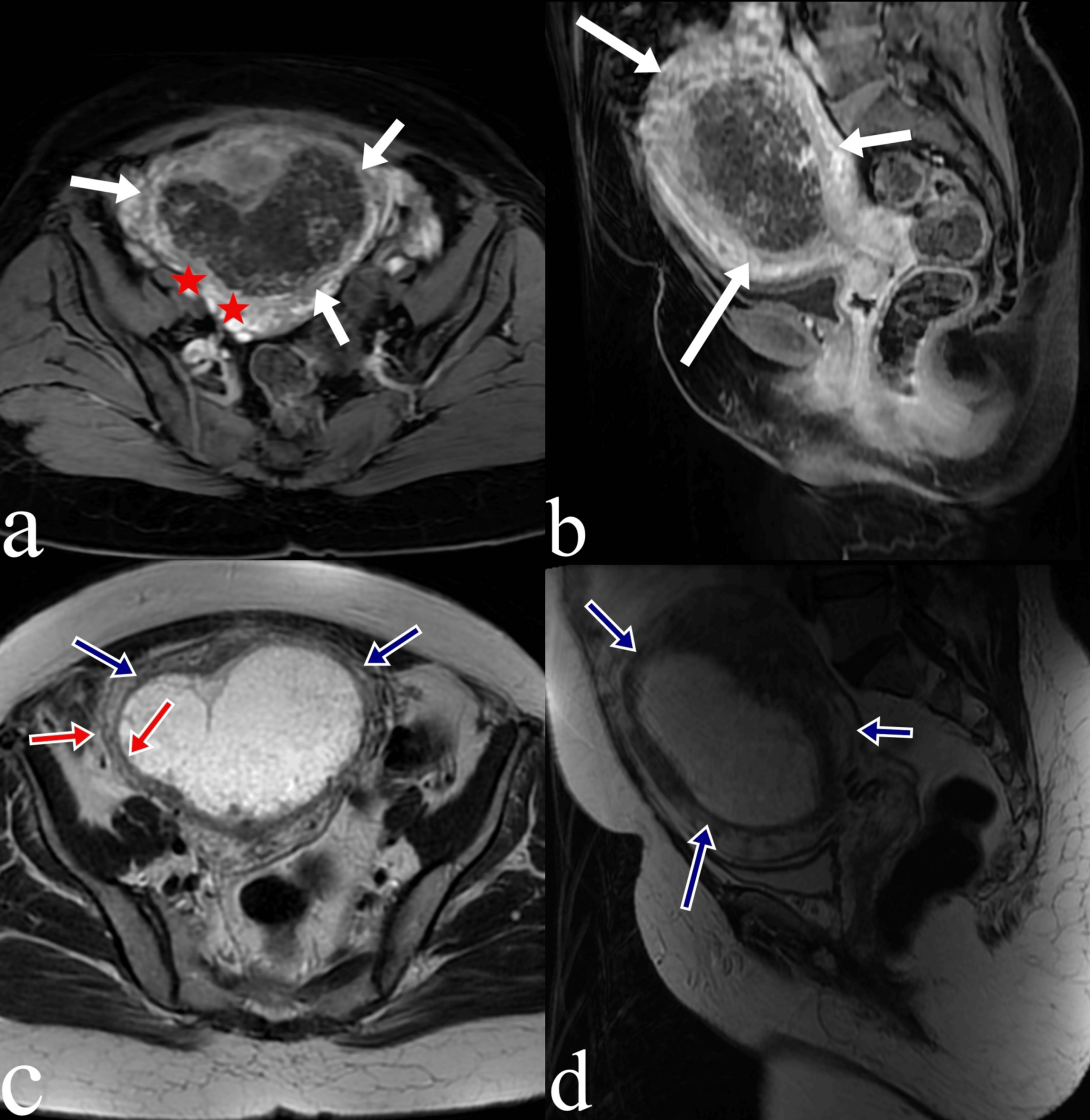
T1- and T2-weighted magnetic resonance images of the mass lesion (a) Axial and (b) sagittal T1-weighted magnetic resonance images show a mass lesion with a more vascular pattern compared to the myometrium (white arrows). Thinning and invasion of the myometrium are observed in the right half of the fundus (red asterisk). (c) Axial and (d) sagittal T2-weighted images show the mass lesion with hyperintense signal characteristics compared to the myometrium (blue arrows) and thinning of the myometrium in the right half of the fundus (red arrows).
